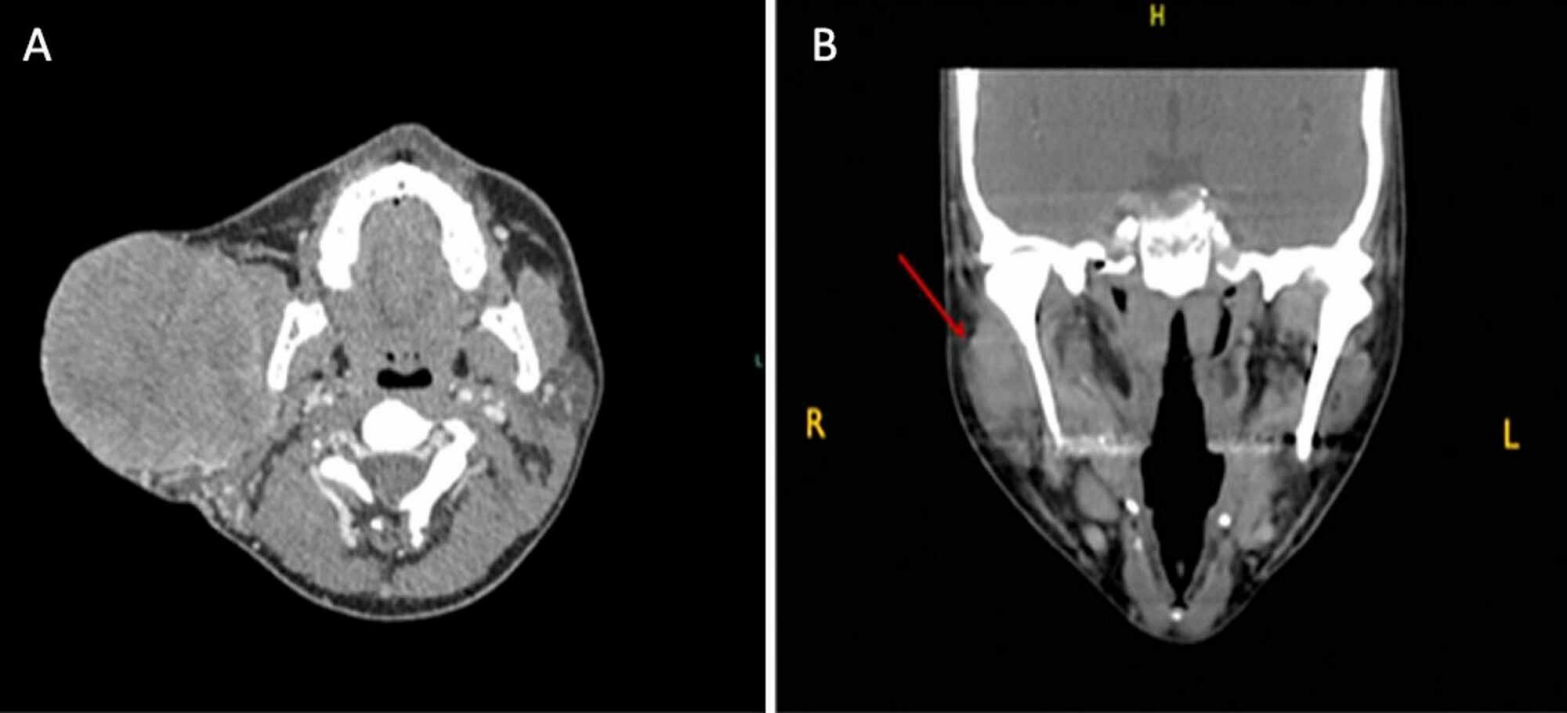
CT head and neck with contrast (A) First case: CT head and neck with contrast showing right parotid gland mass measuring 8.5 × 8.4 × 8.3 cm with central necrosis and mass effect on sternocleidomastoid muscle. (B) Second case: neck CT scan showing poorly demarcated mass (arrow) occupying superficial lobe of right parotid gland.
